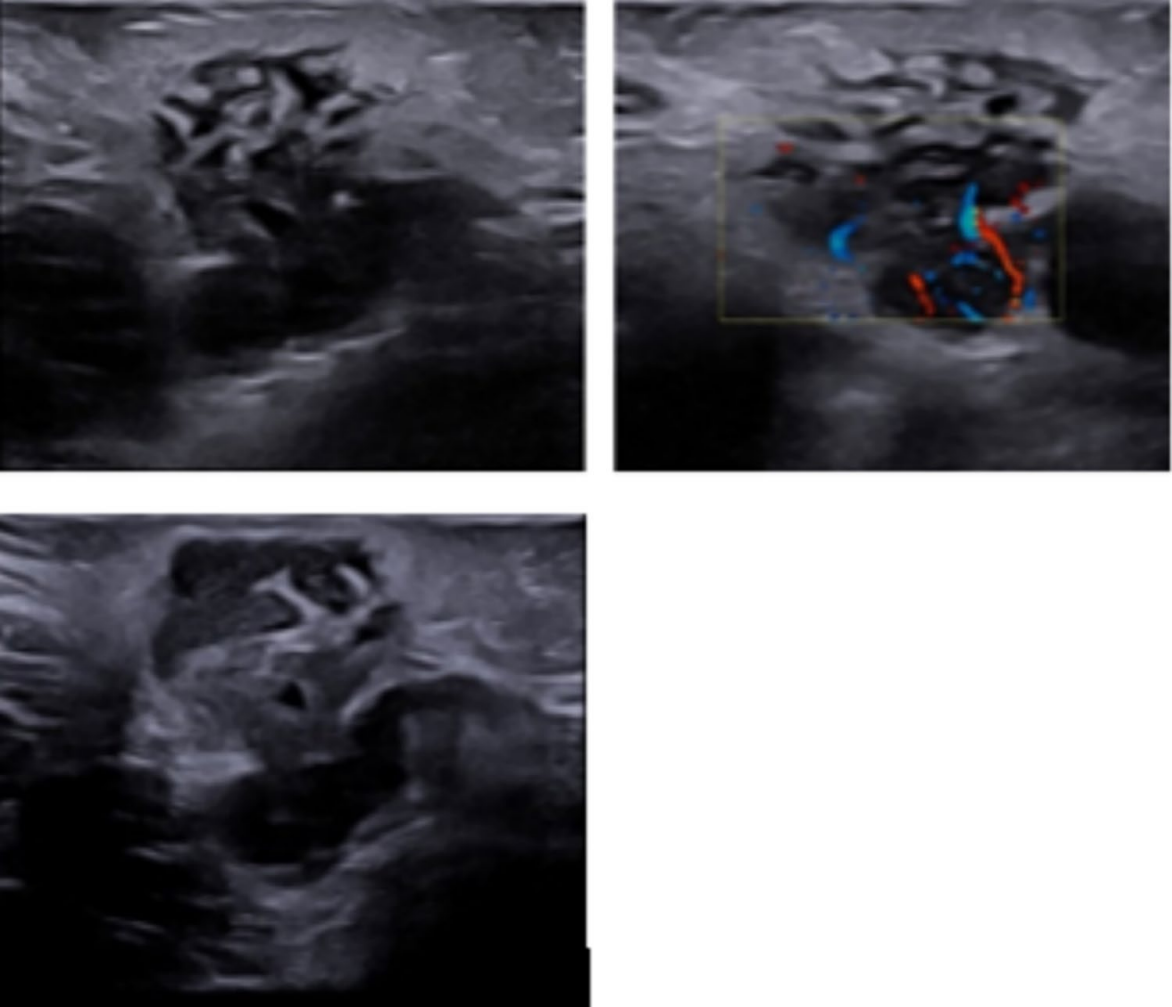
Follow-up 2 months after the disease onset: lymph nodes with reactive characteristics in contact with an inhomogeneous area without Doppler signal referable to necrotic-colliquative cicatricial outcomes; gradual resolution of the inflammation of the soft tissues around the lesion
